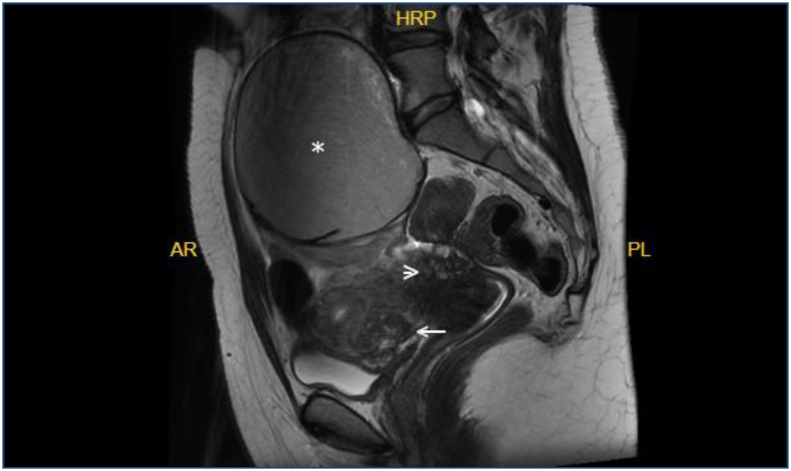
Sagittal T2-weighted imaging. Endometrioma with "T2 shading sign"(asterisk), vesicouterine space and posterior bladder wall endometriosis (arrow), and torus uterinus involvement (arrowhead).
